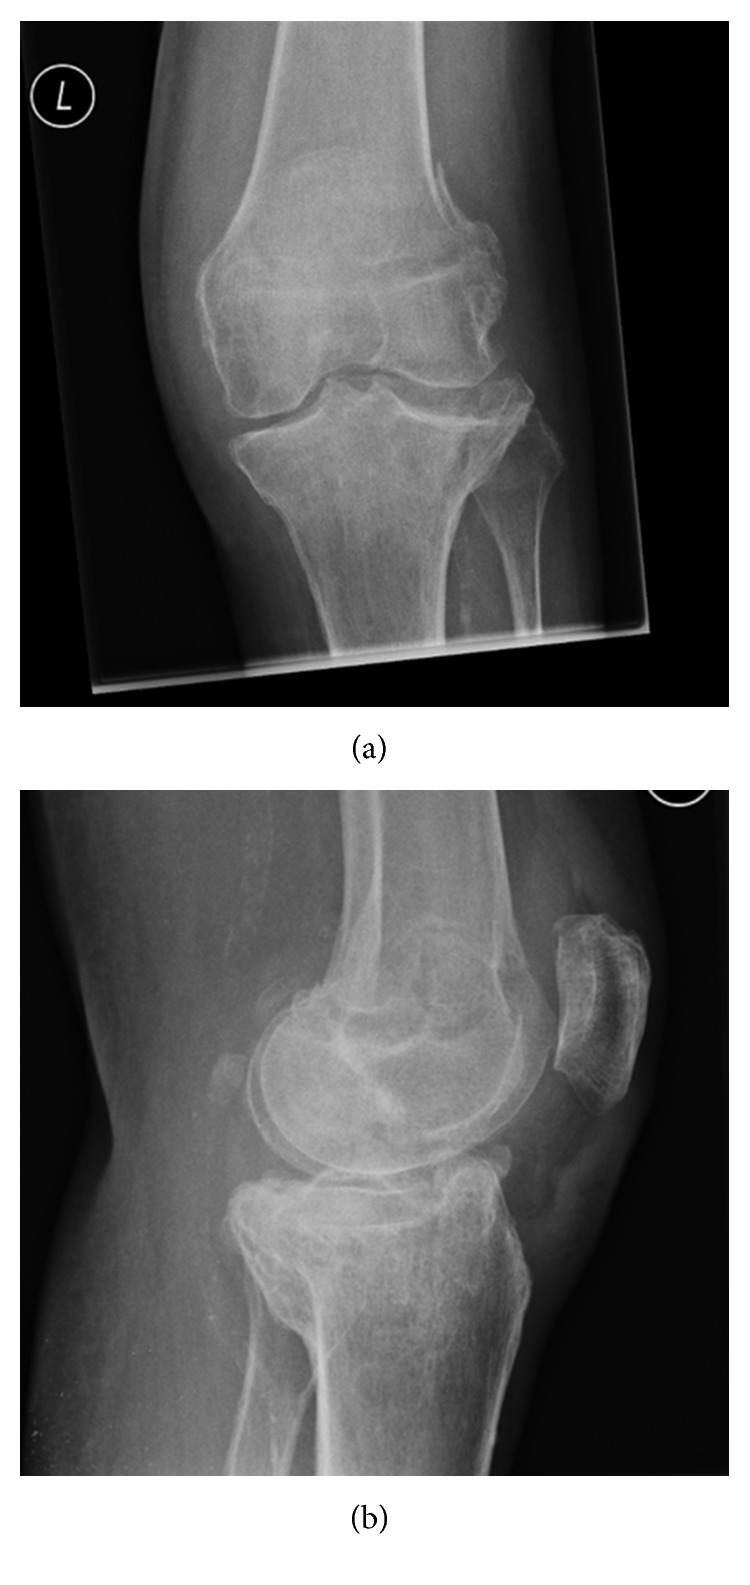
(a) AP and (b) lateral radiographs demonstrating intra-articular femoral and tibial fractures.
